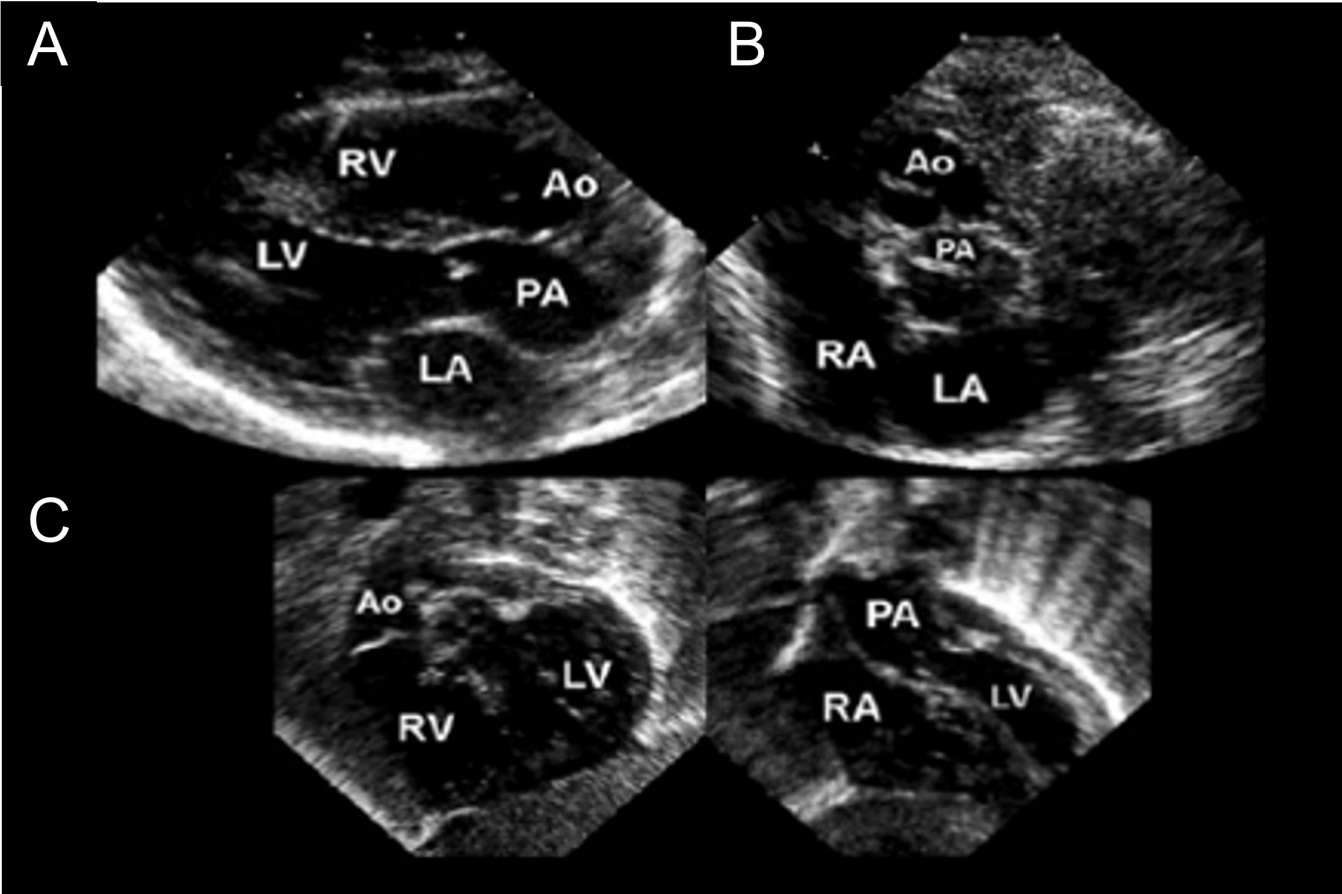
Echocardiography in Dextro (D)-Transposition of the great
